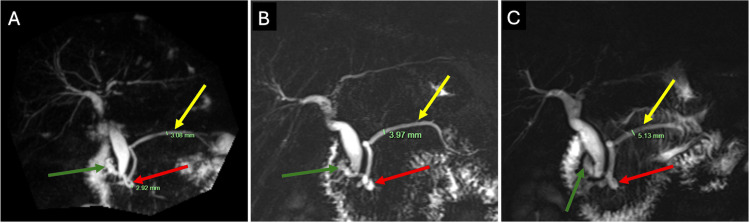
MRCP images demonstrating gradual progressive dilatation of the main pancreatic duct Coronal T2-weighted MRCP maximum-intensity projection images demonstrating progressive dilatation of the main pancreatic duct (yellow arrow), measuring approximately (A) 3 mm in November 2018, (B) 4 mm in September 2019, and (C) 5 mm in February 2020. A santorinicele (green arrow) and ansa pancreatica (red arrow) are also evident. MRCP, magnetic resonance cholangiopancreatography.
